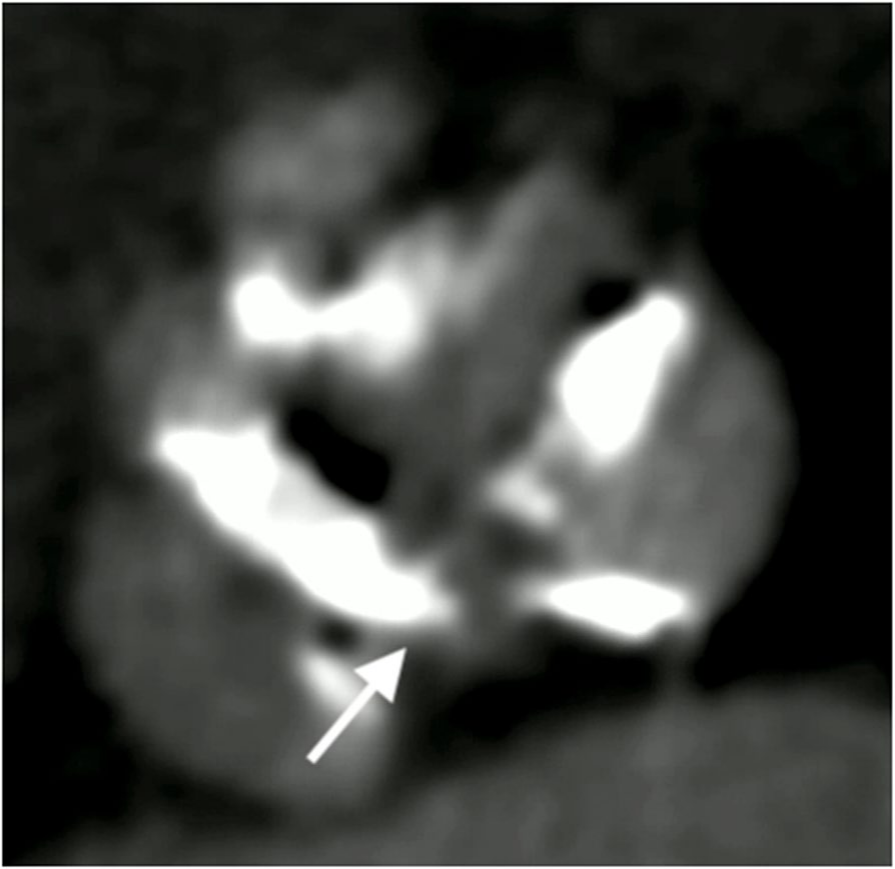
Preoperative computed tomography image showing bulky calcifications on the right coronary cusp (RCC), left coronary cusp (LCC), and non-coronary cusp (NCC). Notably, NCC calcification extends toward the N–L commissure (arrow)
